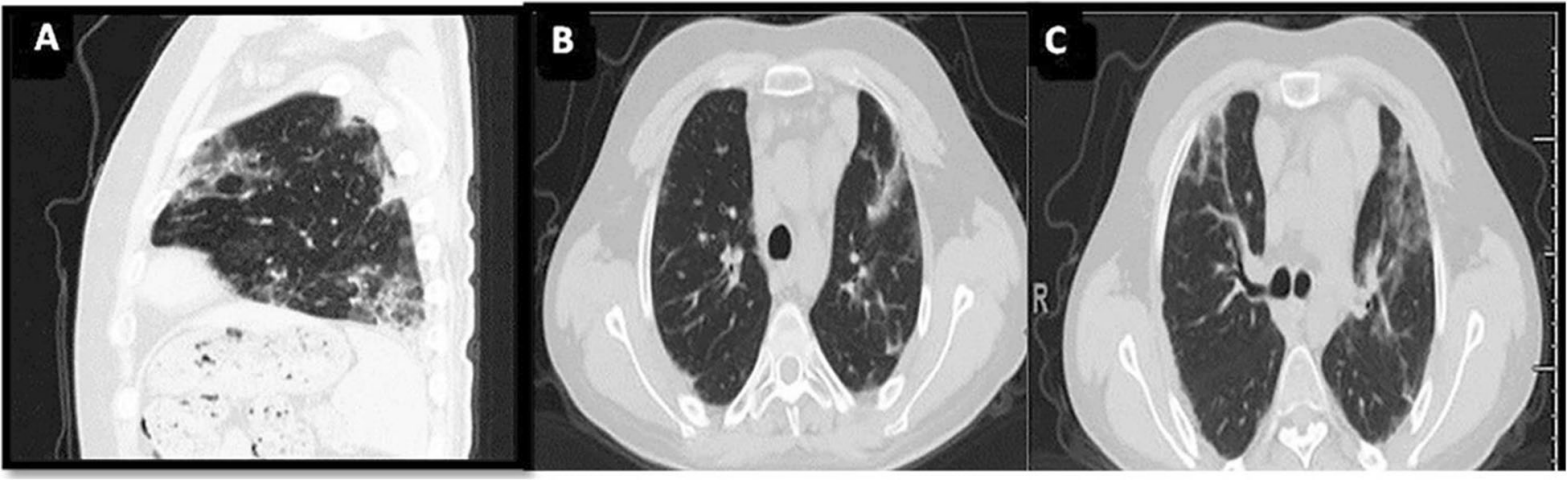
Fourth-week follow-up chest CT of a female patient aged 12 years old with ALL and receiving chemotherapy, who developed COVID-19 infection presenting with dyspnea, tachypnea, and fever. The patient needed O_2_ at the time of presentation. The initial chest CT severity score was 18 and, 1 month later, there were residual consolidation patches, linear opacities, crazy-paving patterns, and linear opacities. Chest CT severity score was 8
